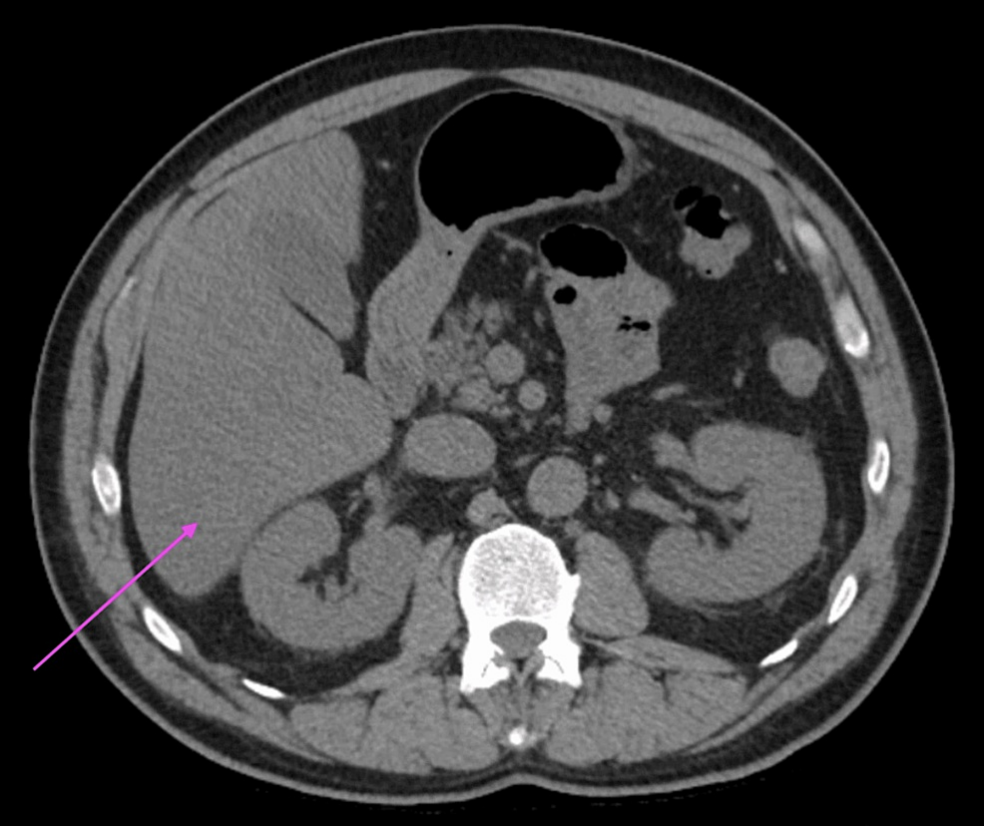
CT abdomen revealed fatty infiltration of the liver (marked by the pink arrow), a small hiatal hernia, and a 1.6-cm right adrenal mass
